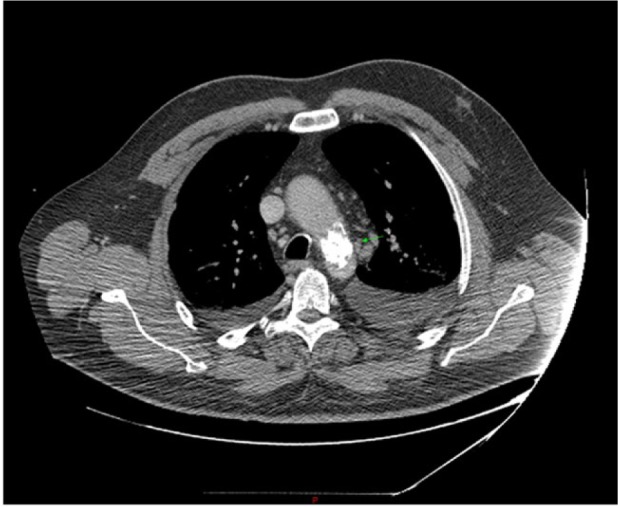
Calcified aortic lesion near aortic arch by computed tomography angiography.
